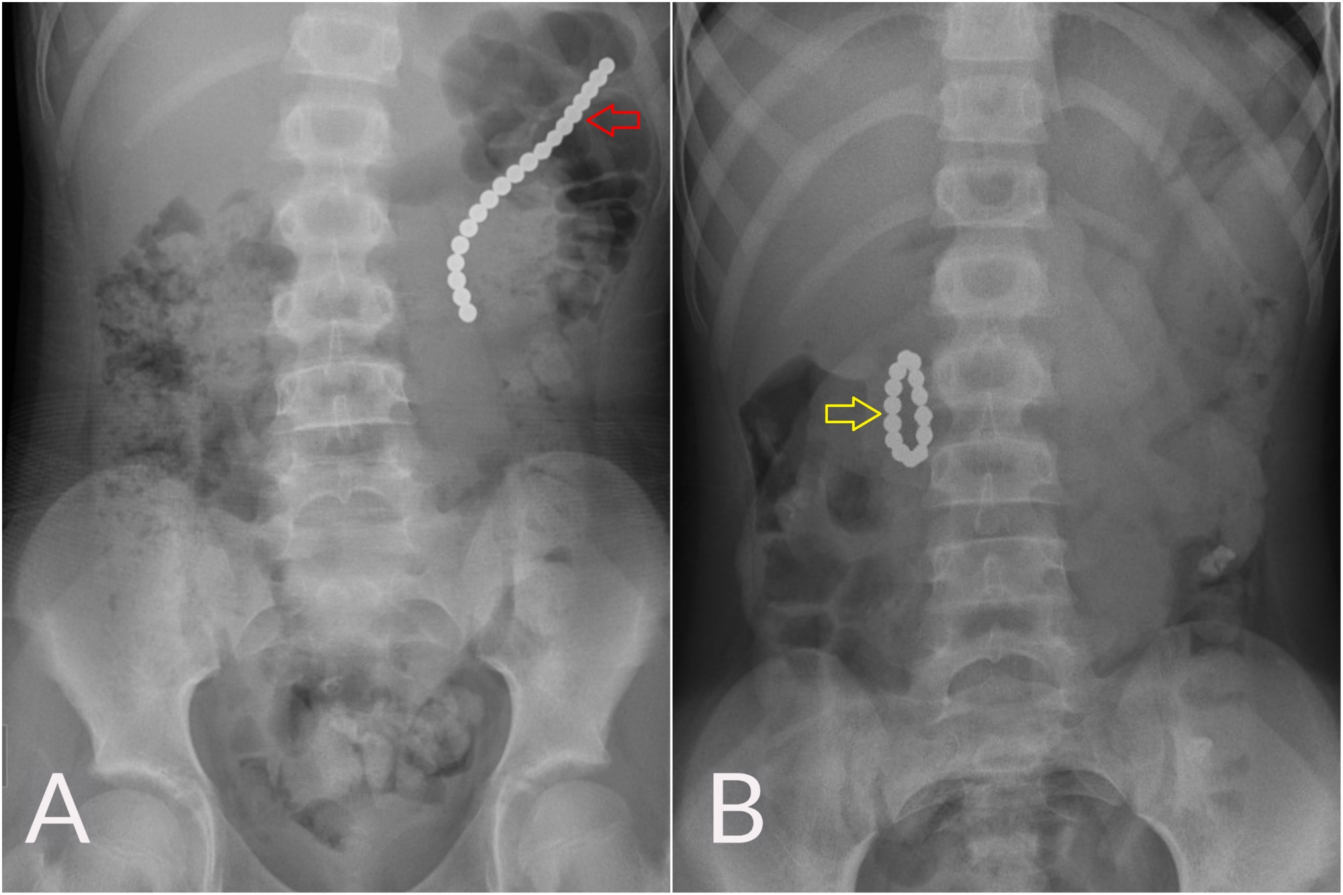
Abdominal x-rays (A) Abdominal x-ray shows a chain of 19 magnetic beads in the stomach (red arrow). (B) Intra-operative abdominal x-ray reveals that the 14 remaining magnets have migrated into the small bowel and formed a ring (yellow arrow)
